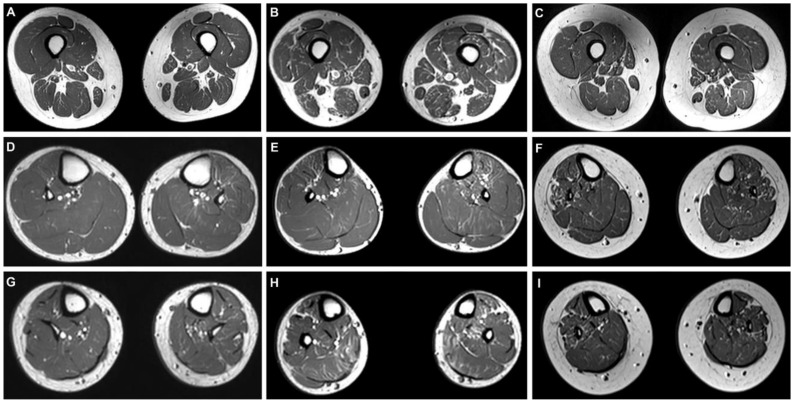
Axial T1-weighted MRIs of the distal thigh (top), proximal (middle) and distal calf (bottom) in FC777 II-2, FC822 II-3, and FC780 II-3. FC777 II-2 (female, 32 years old) shows only minimal fat infiltration in thigh and calf muscles (A,D,G). Both FC822 II-3 (male, 17 years old) and FC780 II-3 (female, 20 years old) show mild fat infiltration in the anterior compartment muscles of the distal thigh (B,C). FC822 II-3 shows moderate fat infiltration in the soleus muscle at the distal calf and mild fat infiltration in the anterior compartment of the proximal and distal calf (E,H). FC780 II-3 shows mild fat infiltration in lateral compartment muscles of the proximal and distal calf (F,I).
